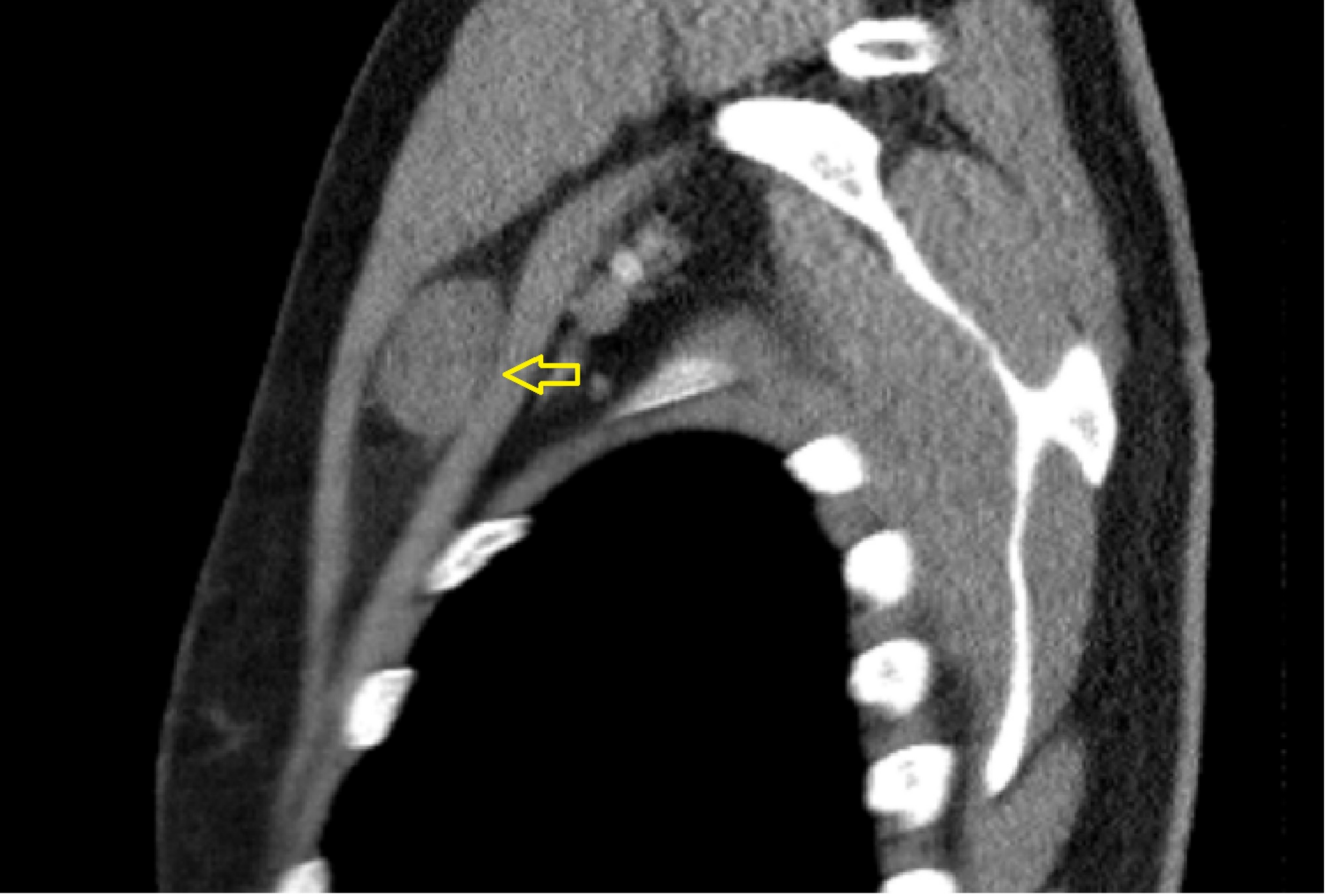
Saggital CT of the chest (with contrast) Redemonstrated well-defined, rounded, soft tissue density (arrow) between the left pectoralis major and minor muscles. CT: computed tomography
